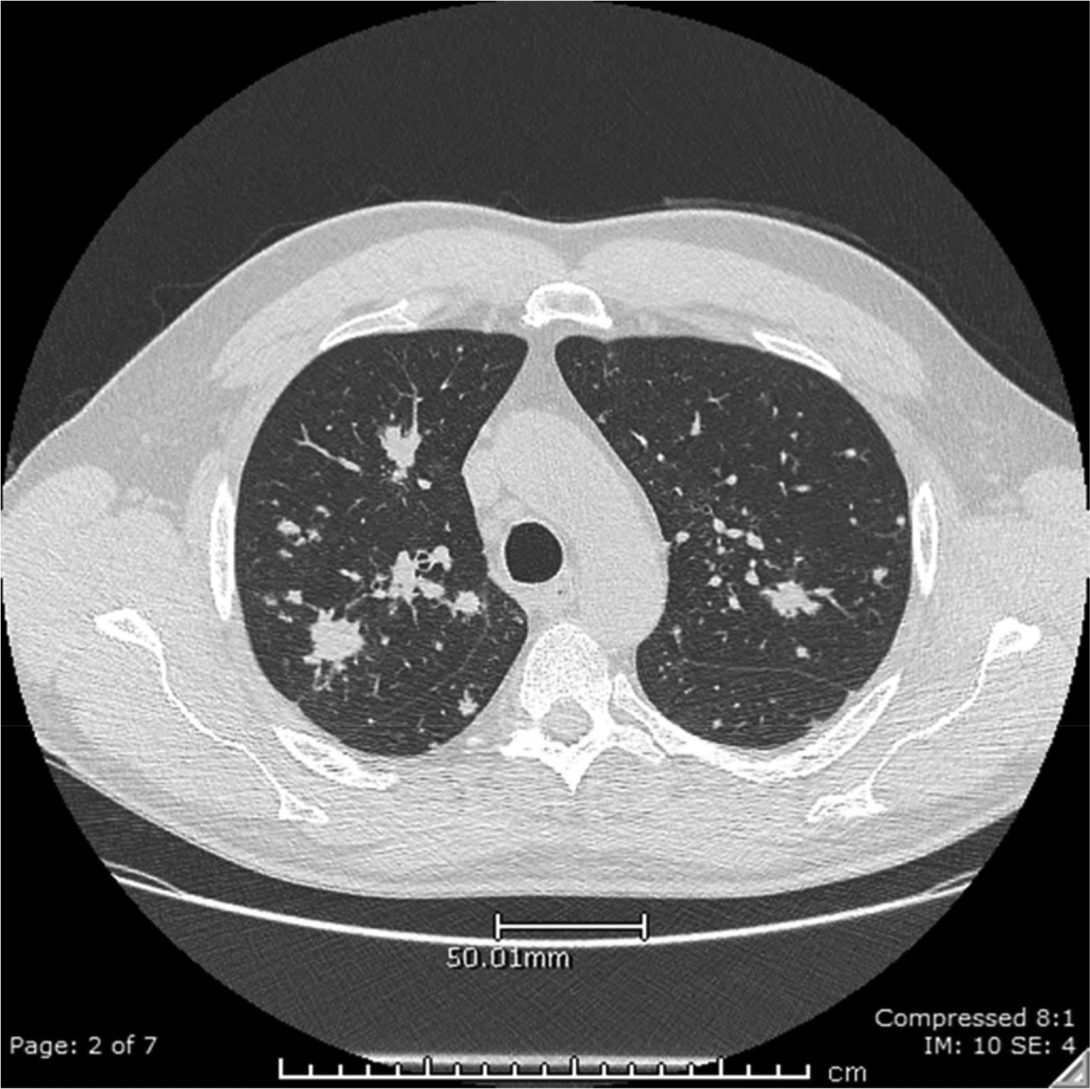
High resolution computed tomography demonstrating bilateral interstitial opacities.
